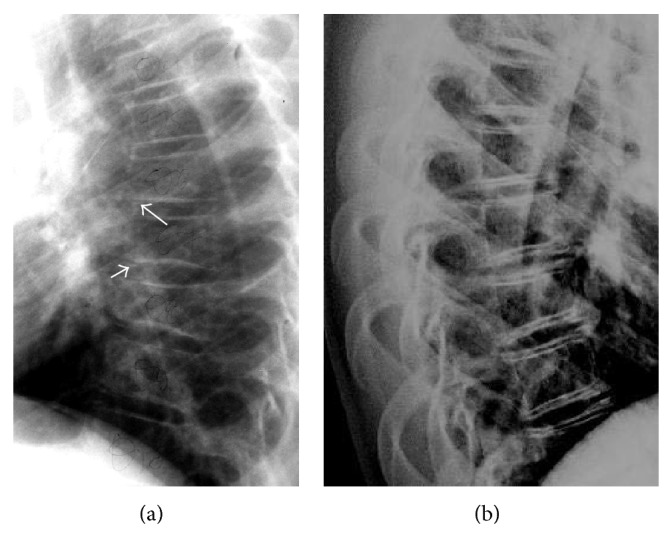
Lateral radiographs of the thoracic spine: (a) three months, (b) two years after bisphosphonate treatment ceased. In (a) there was osteopenic appearance, decreased height, and mild end-plate sclerosis of the thoracic vertebral bodies, whereas faint ossifications of the anterior endplates were seen (arrows). In (b) a typical “bone in bone” appearance of thoracic vertebral bodies was apparent.
